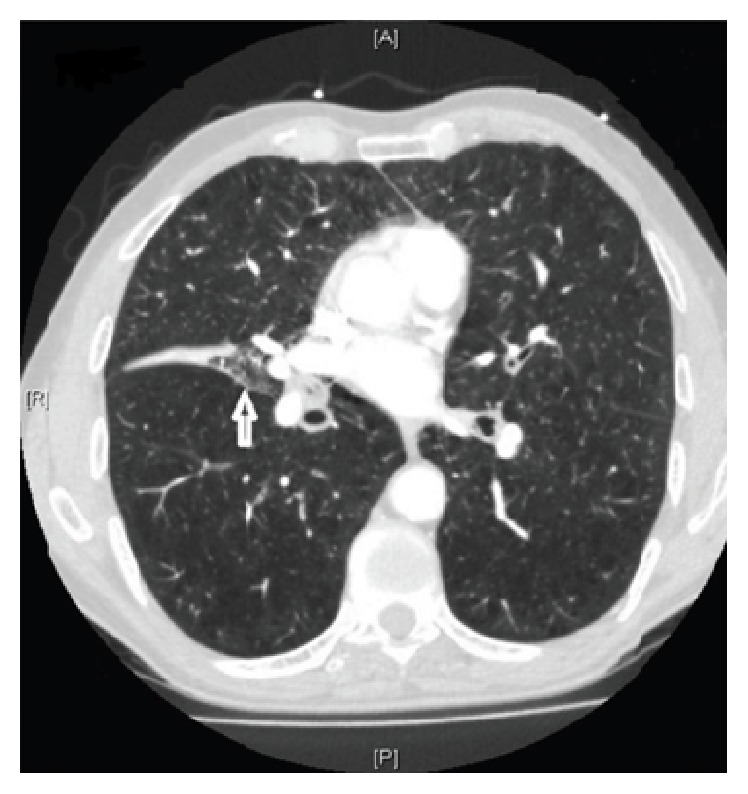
CT-angiogram of the lung showing severe emphysema and near complete collapse of right middle lobe.
